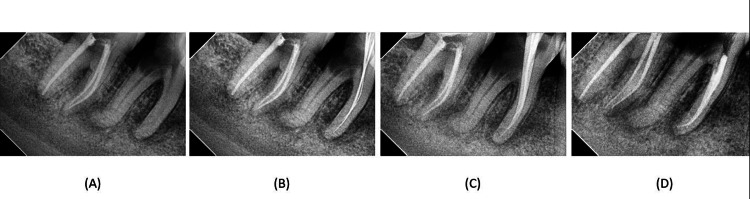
(A) Preoperative radiograph of tooth number 46; (B) working length of tooth number 46; (C) master cone selection of the mesial root of tooth 46; (D) obturation of the mesial root of tooth 46
